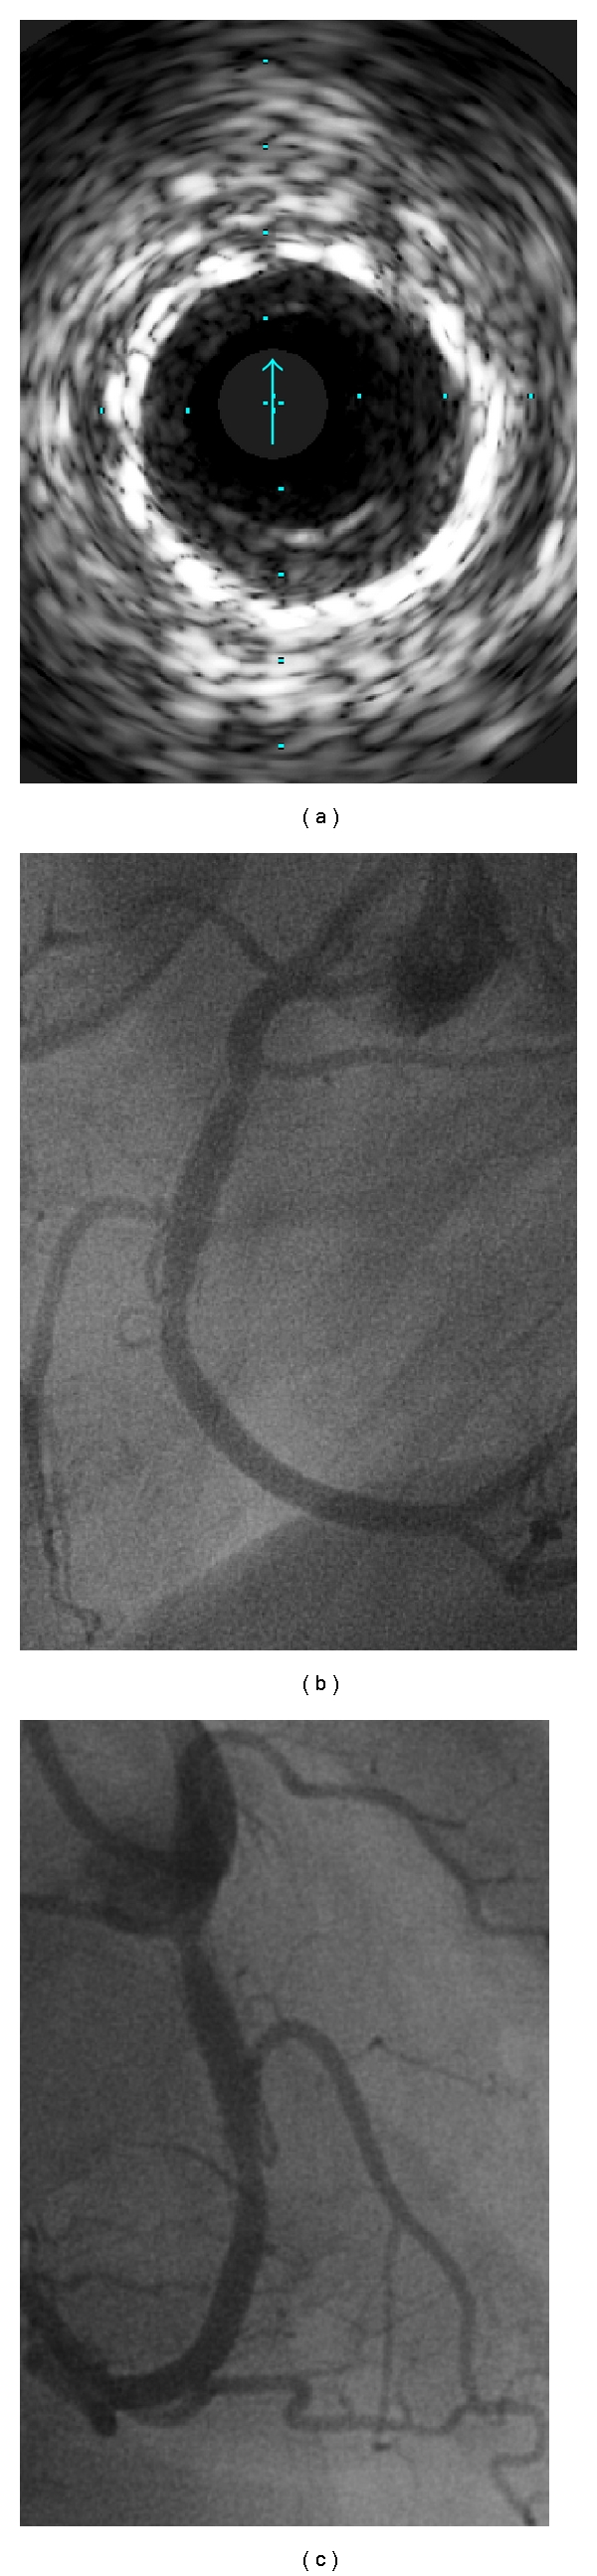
Intravascular ultrasound (IVUS) (a) shows moderate hyperplasia of the intima and well-apposition of the PTFE-covered stent to the first implanted stent. Right coronary angiography in caudal left anterior oblique (b) and in right anterior oblique projection (c) shows the channel between the right coronary artery and the side branch. Both IVUS and right coronary angiography were done 11 months after the incident.
